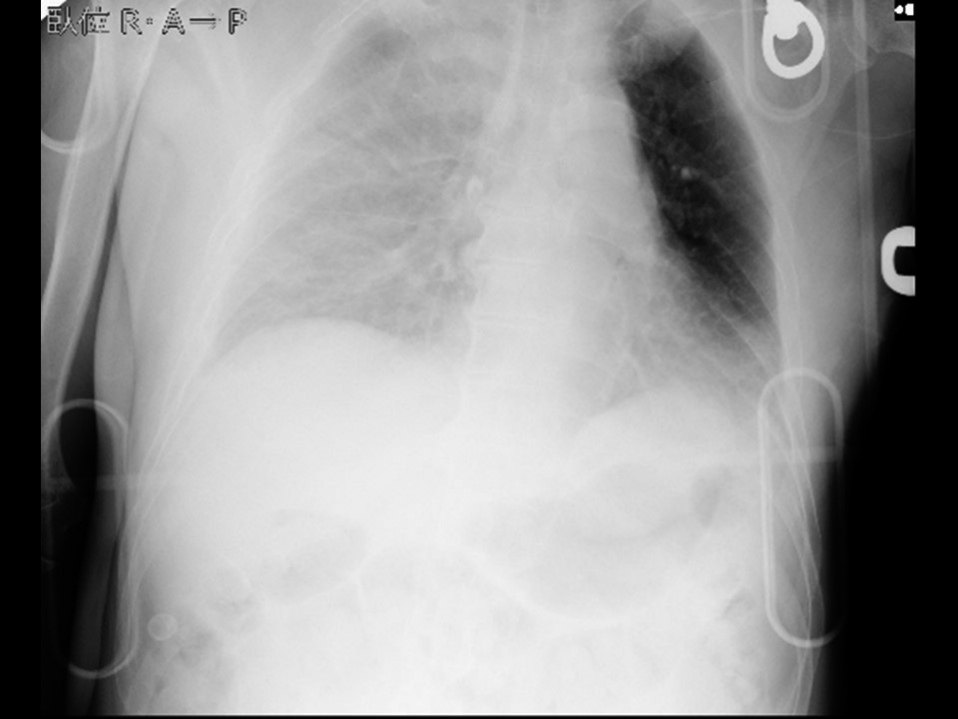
Chest X-ray on arrival The X-ray suggested right hemothorax.
